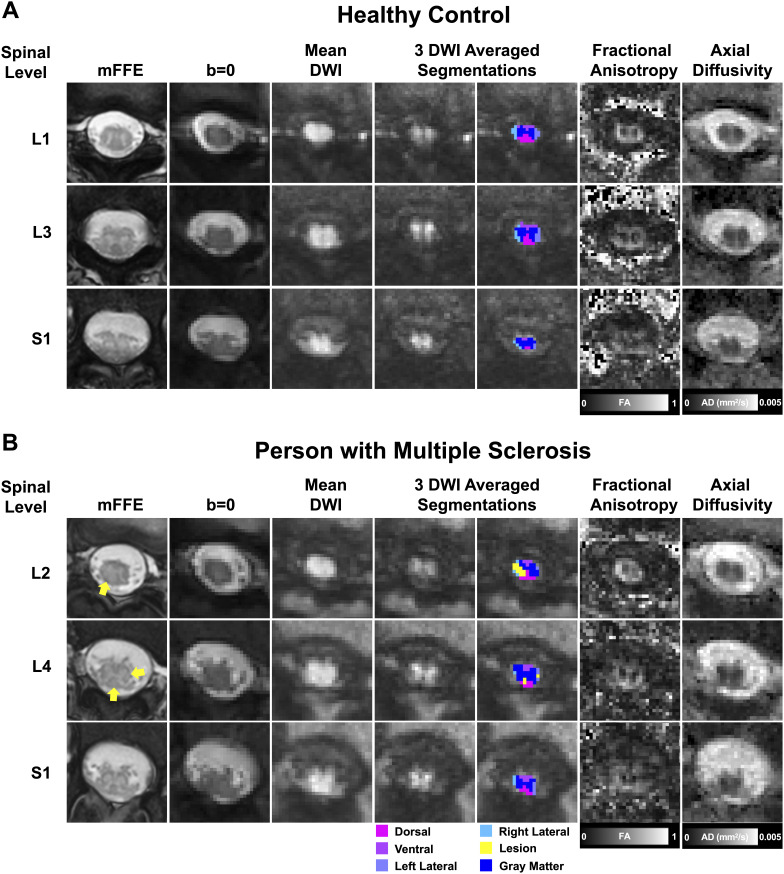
Example data for one healthy control (A, 26-year-old female) and one person with multiple sclerosis (B, 42-year-old female). Shown are the anatomical multi-echo, gradient echo (mFFE) images, image with no diffusion-sensitizing gradients (b = 0), mean diffusion-weighted image (DWI), three DWI slices averaged with good gray matter (GM) contrast without (left) and with (right) GM, white matter columns, and lesions overlaid, and the fractional anisotropy (FA) and axial diffusivity (AD) quantitative maps. All images are shown for three slices corresponding to spinal levels throughout the lumbar enlargement. Lesions are highlighted by the yellow area on the anatomical slices.
